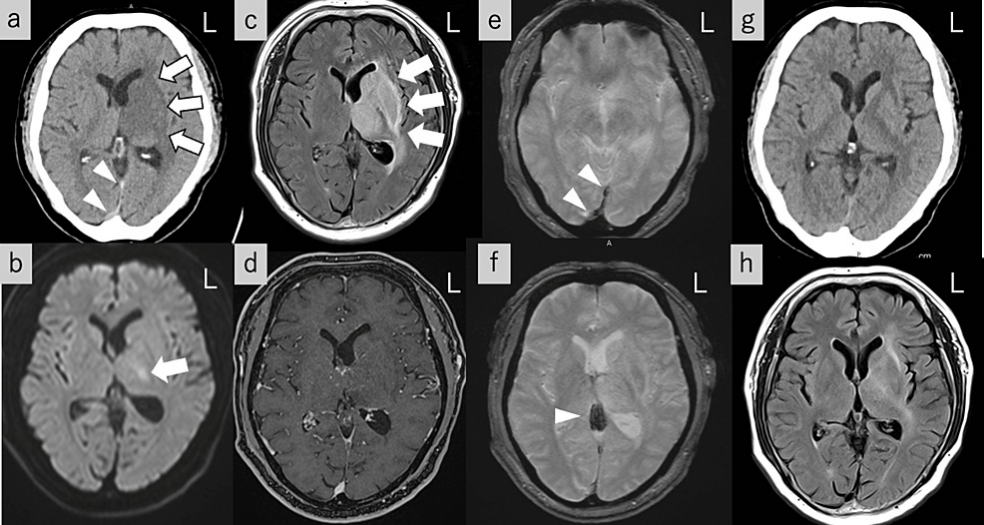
Diagnostic and follow-up findings of the present case. a: Axial computed tomography (CT) showed edematous changes in the left thalamus to basal ganglia (arrows) and high-density lesion in the straight sinus (arrowheads). b: Diffusion-weighted imaging showing high-intensity lesion confined to the basal ganglia (arrow). c: Fluid-attenuated inversion recovery (FLAIR) showed broad high-intensity lesions with edematous changes in the left thalamus and basal ganglia (arrows). d: Contrast-enhanced magnetic resonance imaging showed no brain tumor in the left thalamus and basal ganglia. e,f: T2*-weighted imaging (T2*WI) showed low intensity suggesting thrombus in the vein of Galen to straight sinus (arrowheads). g: Follow-up CT showed improvement of edematous changes in the left thalamus to basal ganglia. h: Follow-up FLAIR showed improvement of the left thalamus and basal ganglia lesion.
